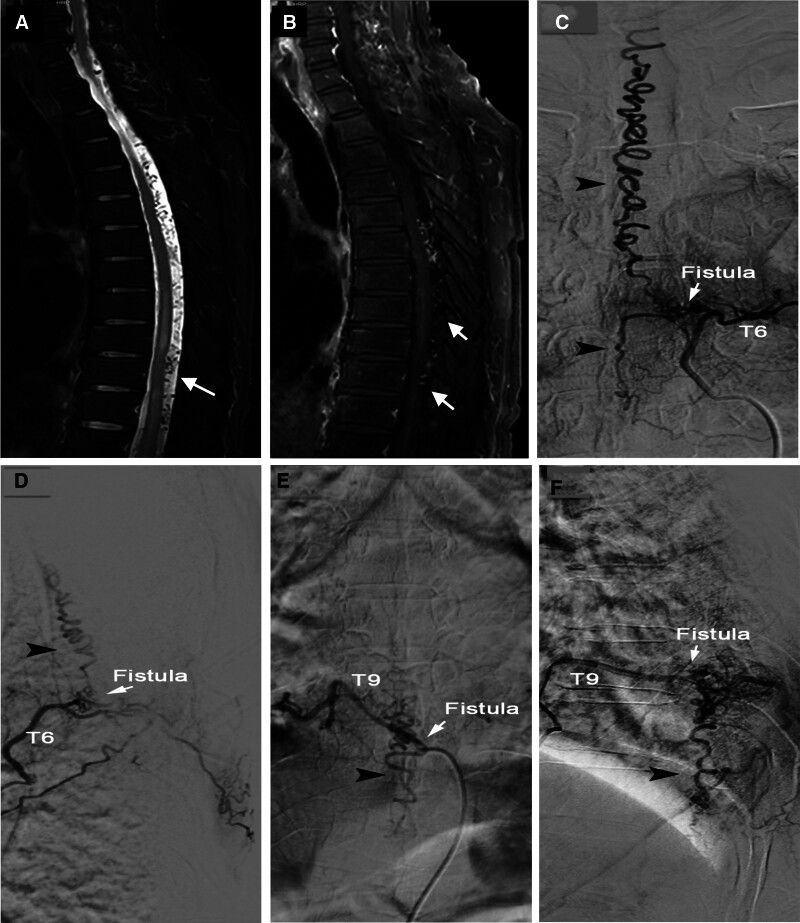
Preoperative spinal T1 and T2 weighted magnetic resonance imaging (MRI) and angiography. (A) A T2 weighted image showing spinal cord edema and tortuous vessels on the surface of the spinal cord (white arrow). (B) A T1 weighted image showing spinal cord edema and tortuous vessels on the surface of the spinal cord (white arrow). (C, D) The frontal and lateral views of the T6 spinal dural arteriovenous fistula (white arrow) and draining vein (black arrow). (E, F) The frontal and lateral views of the T9 spinal dural arteriovenous fistula (white arrow) and draining vein (black arrow).
